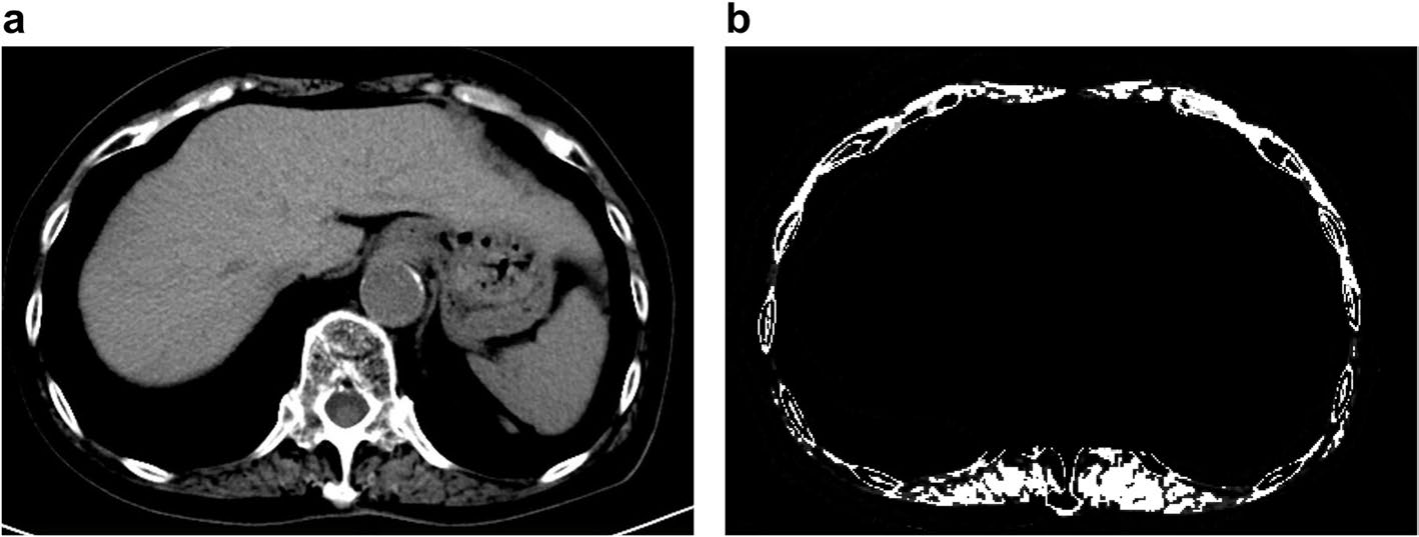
A transverse CT image at the thoracic level T12. a The original CT image, b Total muscle area was identified by MATLAB, including the erector spinae, latissimus dorsi, external and internal oblique, rectus abdominis, and external and internal intercostal muscles
